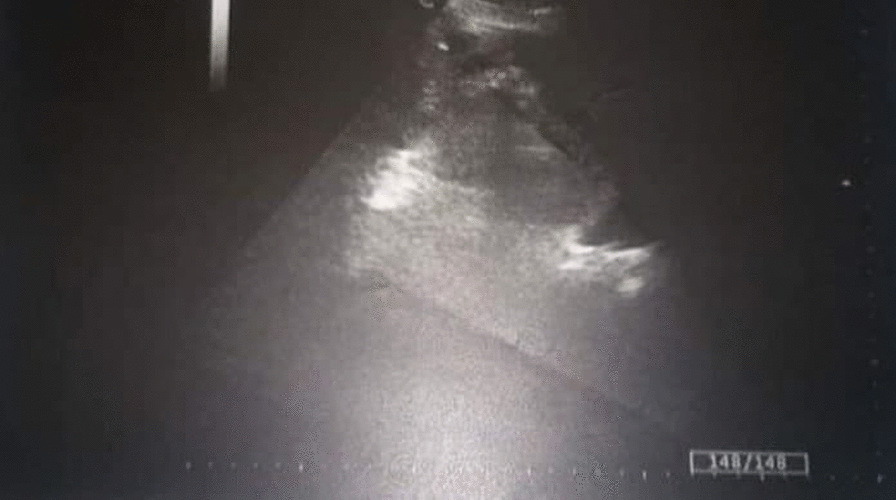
Abdominal Ultrasound showing an accumulation of intestinal loops in the epigastric area
